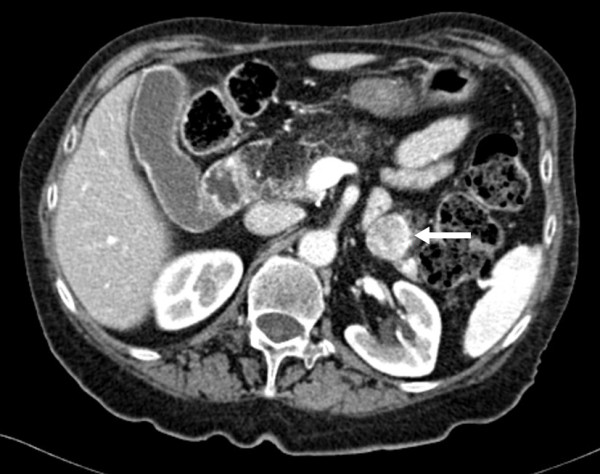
Abdominal enhanced multidetector-row computed tomography (MDCT). A low-density 2x3 cm mass between the body and tail of the pancreas, showing intense contrast enhancement (arrow).
